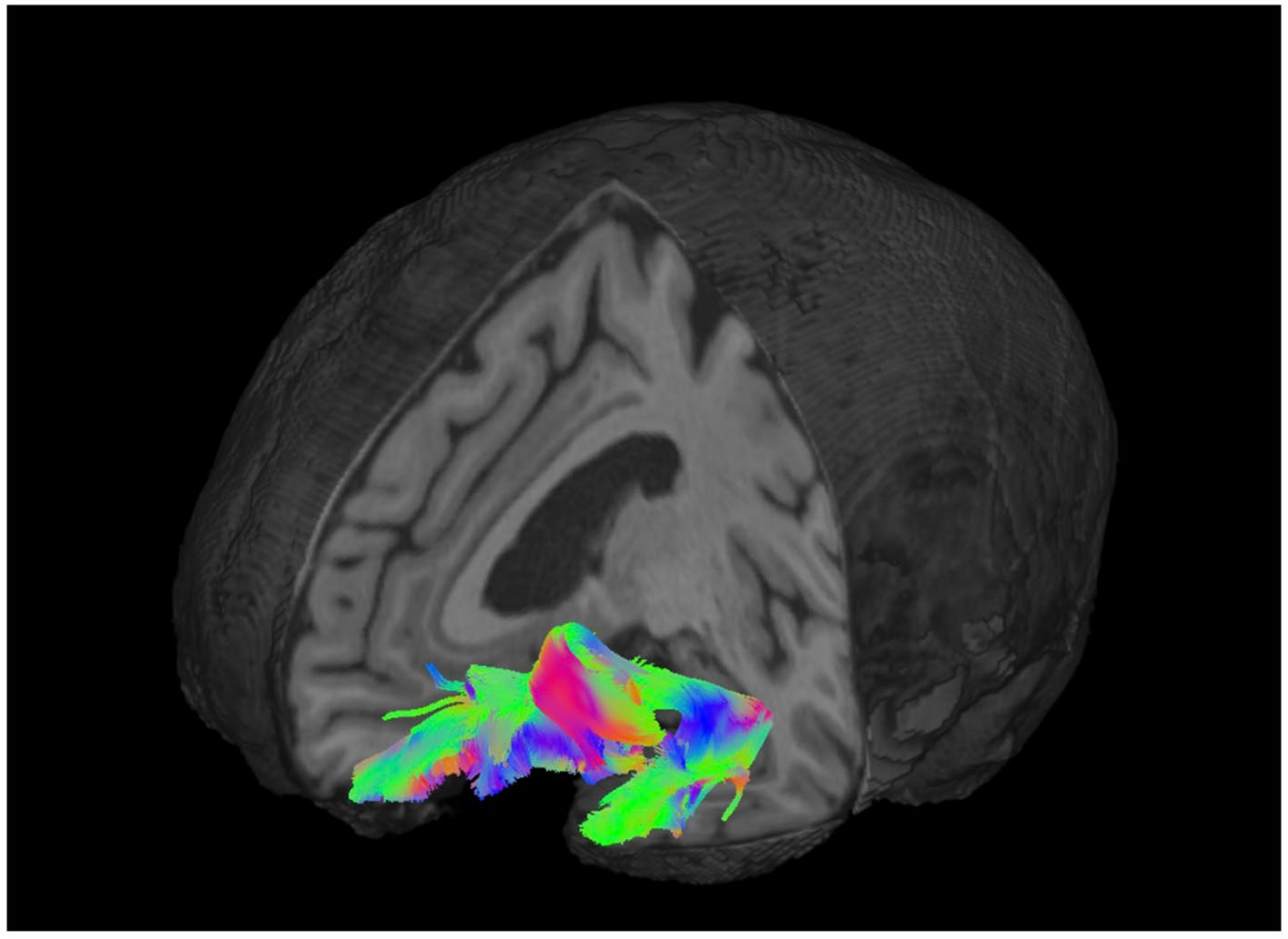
Tractography of the left uncinate fasciculus (UF). Three-dimensional representational reconstruction of the left UF overlaid on a T1-weighted anatomical image. Fiber pathways are color-coded by white matter diffusion direction (red = left–right, green = anterior–posterior, blue = superior–inferior).
